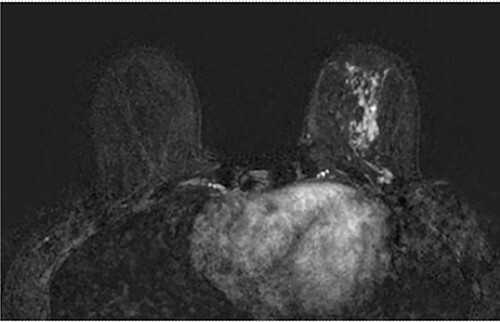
Axial fat-saturated post contrast Magnetic Resonance Imaging (MRI) of the breasts. There is extensive stippled non mass like enhancement throughout the inferior and central left breast with type 1 benign kinetics in keeping with DCIS.
